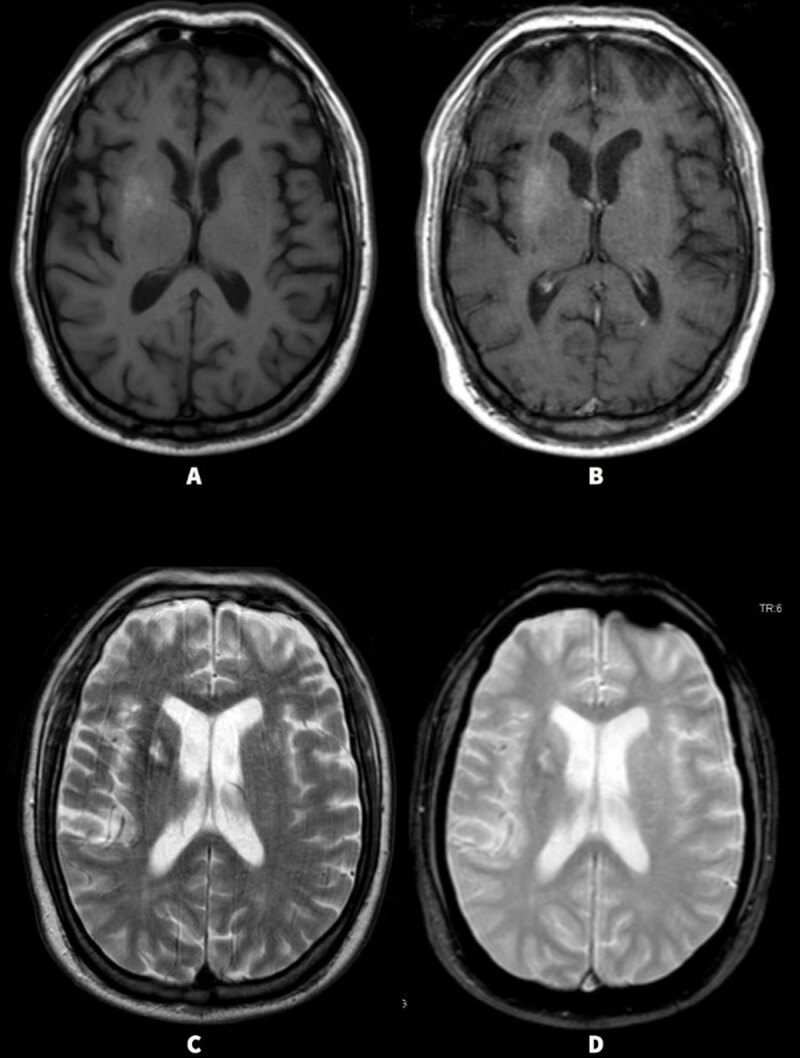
(Case 2) Initial MRI brain images showing T1 hyperintensity in the right basal ganglia (A) with minimal contrast enhancement (B). In the upper lateral aspects of the right caudate, there was a rim of T2 hypointenisty surrounding a central core of T2 hyperintensity (C). The rim had mixed GRE susceptibility (D). There was no evidence of any edema surrounding the right basal ganglia lesion.
